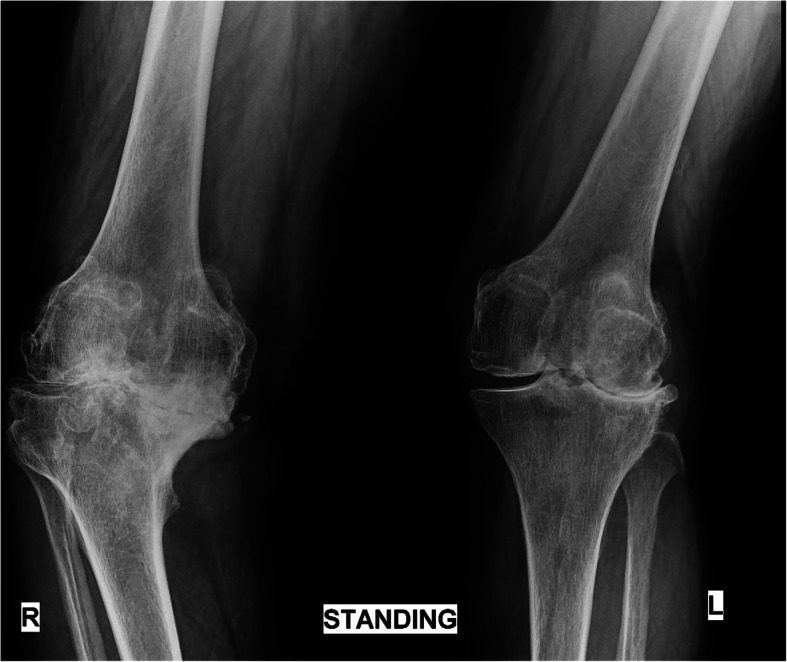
X-ray of a windswept deformity with combined varus and valgus knees
